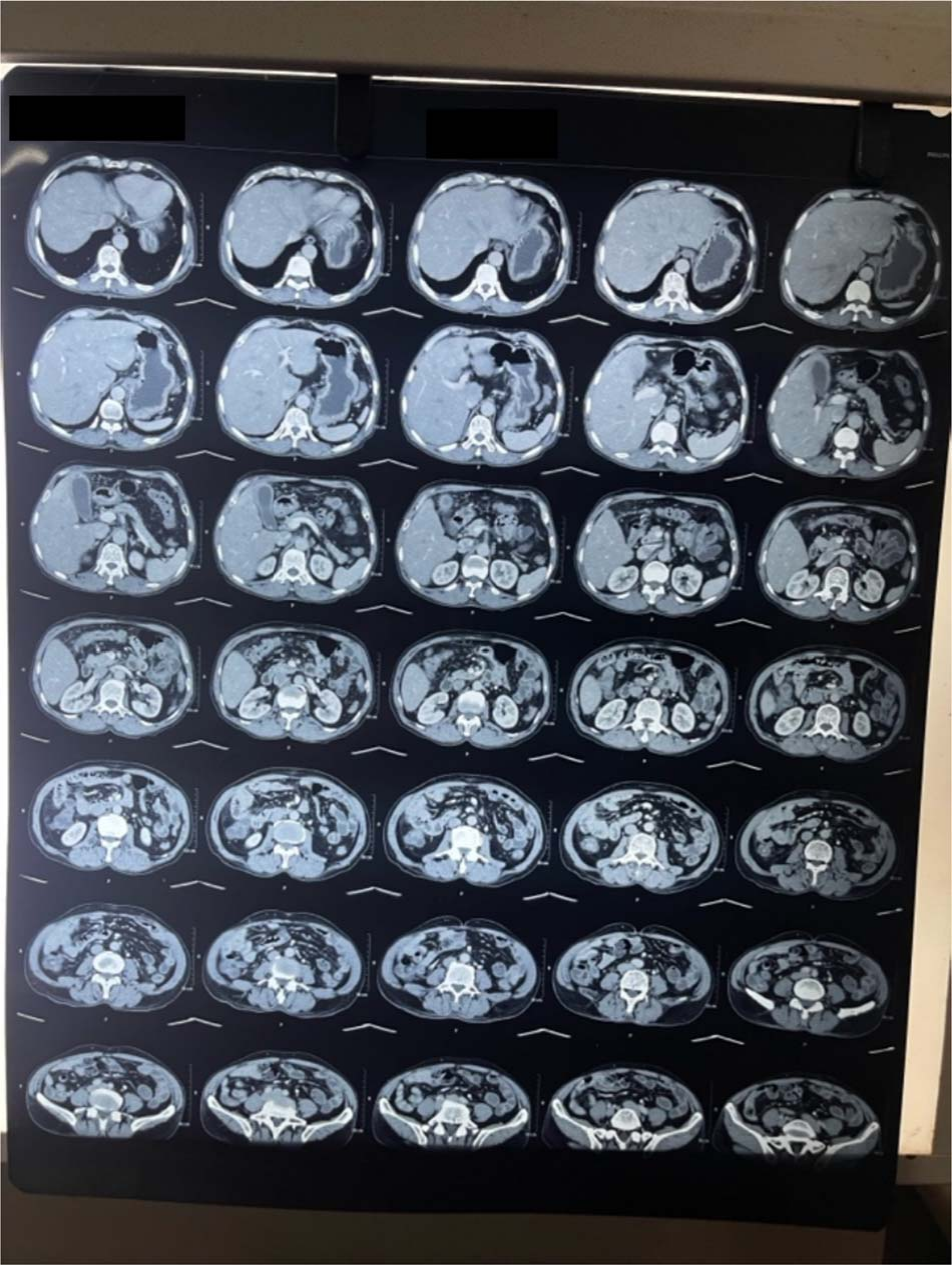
CT abdomen and pelvis transverse cut shows thickened GB wall.
